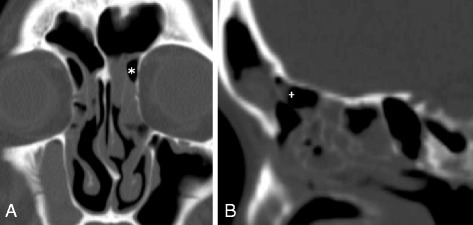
CT images of a patient with frontal sinusitis. a Coronal CT showing a left FC3 (*). b Sagittal CT showing an FBC (+)
